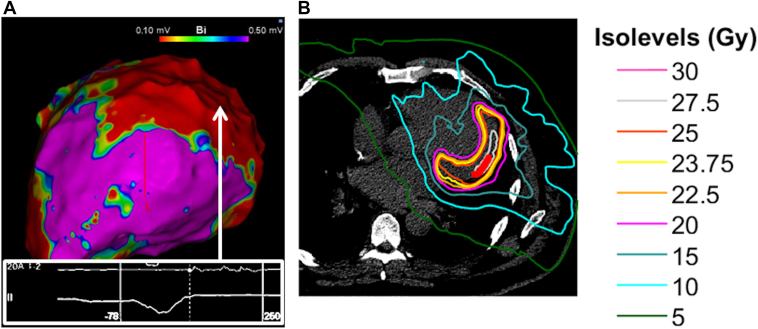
A: An epicardial voltage map obtained with PENTARAY during the fifth catheter ablation procedure (left lateral view). Late potentials were observed in the basal-lateral left ventricular wall. B: An axial computed tomography scan slice displaying the stereotactic arrhythmia radioablation treatment planning. The substrate of ventricular tachycardia in the basal-lateral left ventricular region received 25 Gy.
